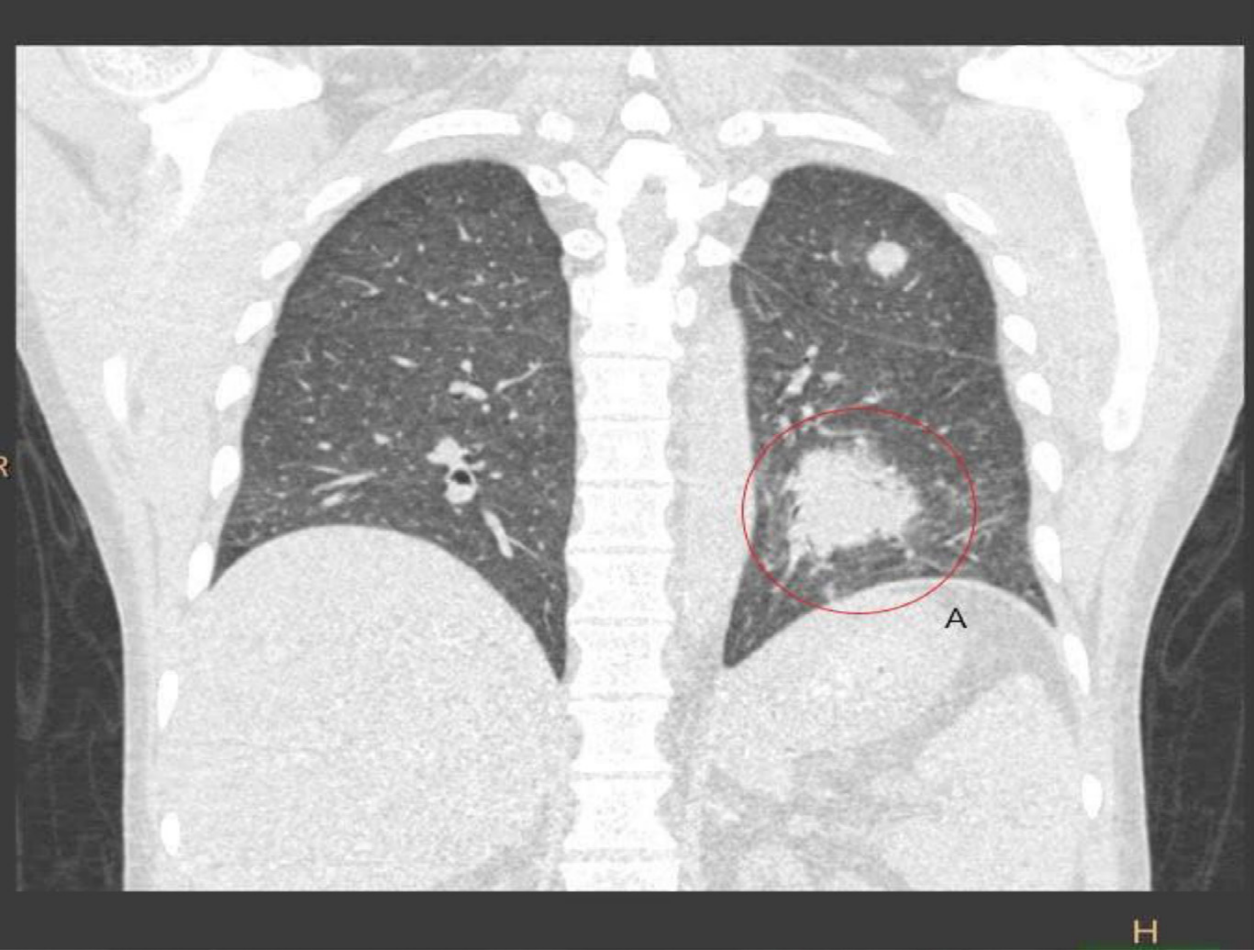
Coronal section of computed tomography scan showing multiple nodules and mass-like consolidations in the left lung. A encircles the largest consolidation.
